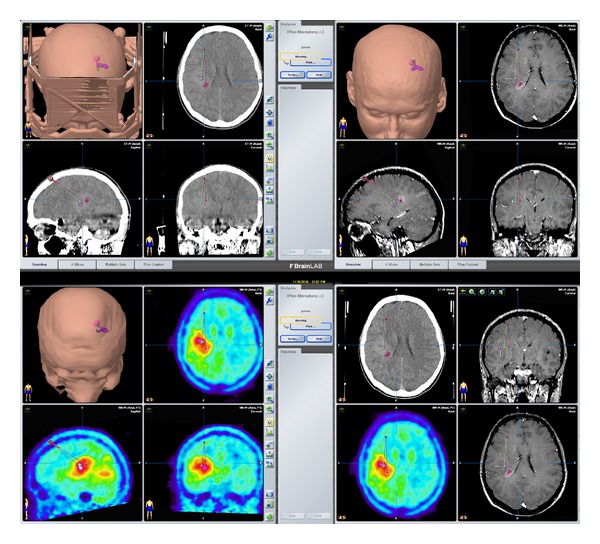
Presurgical navigational overlay of CT, MRI, and FDG-PET in a patient prepared for a stereotactic biopsy. The CT images showed only minor changes, the T1 weighted contrast-enhanced MRI showed a very small contrast affine region but a large hypermetabolic lesion in the left parietal brain was revealed by PET, helping to define the best biopsy aim and trajectory.
